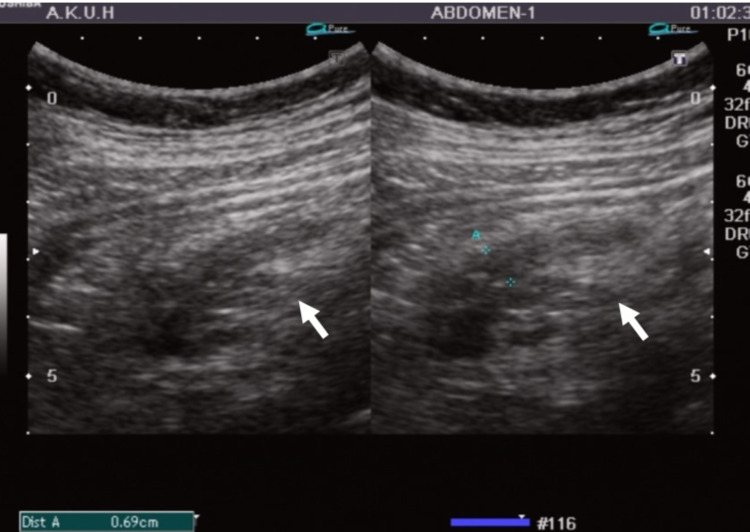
Grade 2 PFES. 13 years with history of lower abdominal pain. Ultrasound of the right iliac fossa demonstrates acute appendicitis with transverse dimension of 0.69 centimeters (A). Periappendiceal fat echo sign (PFES) is seen (white arrow); however, the underlying vessels and muscles can be visualized.
